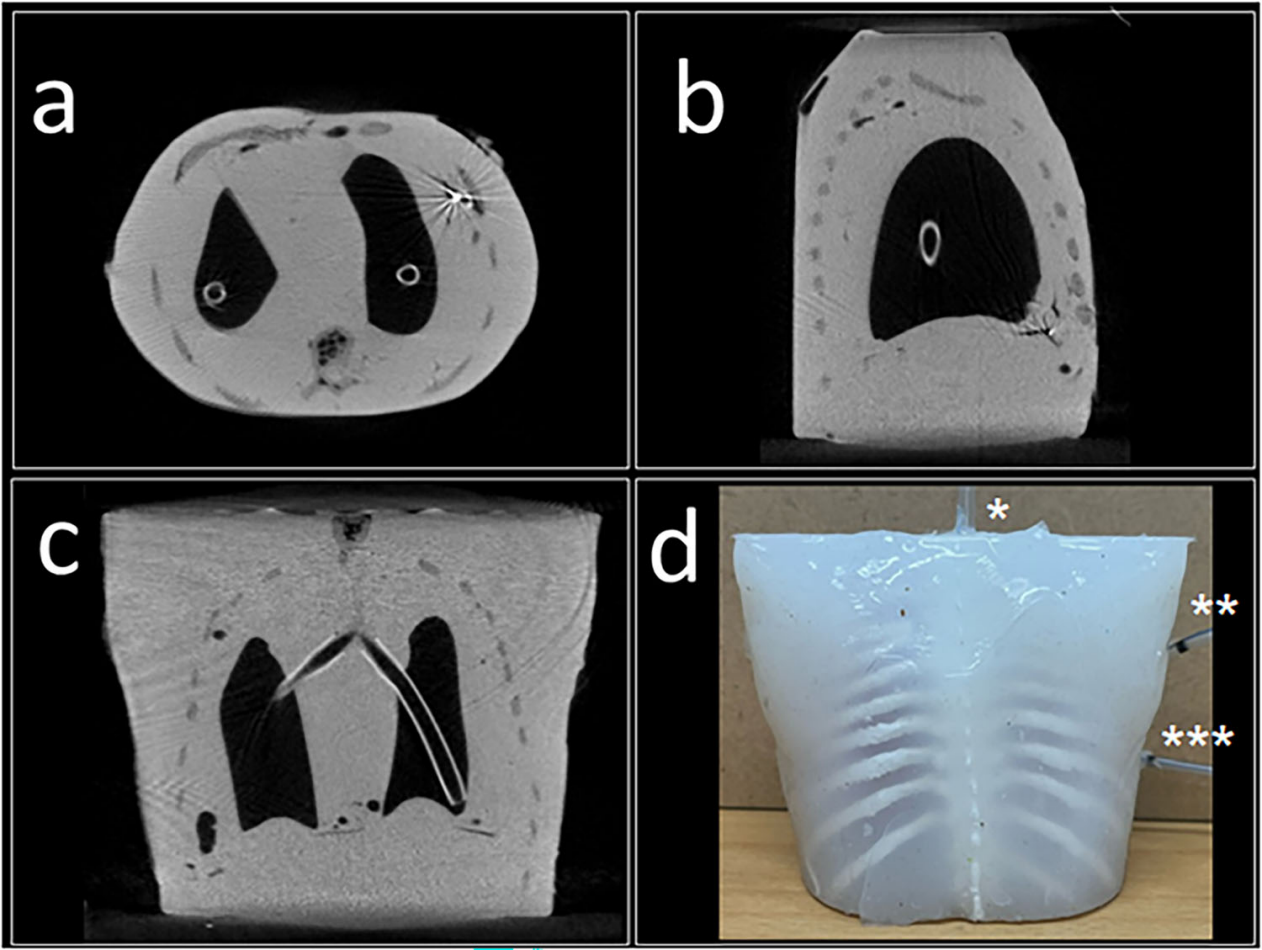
The high‐fidelity 3D‐printed model; (a) CT scan Axial plane of the model, (b) Sagittal plane, (c) Coronal plane, (d) Picture of the model with the tubes attached. [Color figure can be viewed at wileyonlinelibrary.com]
